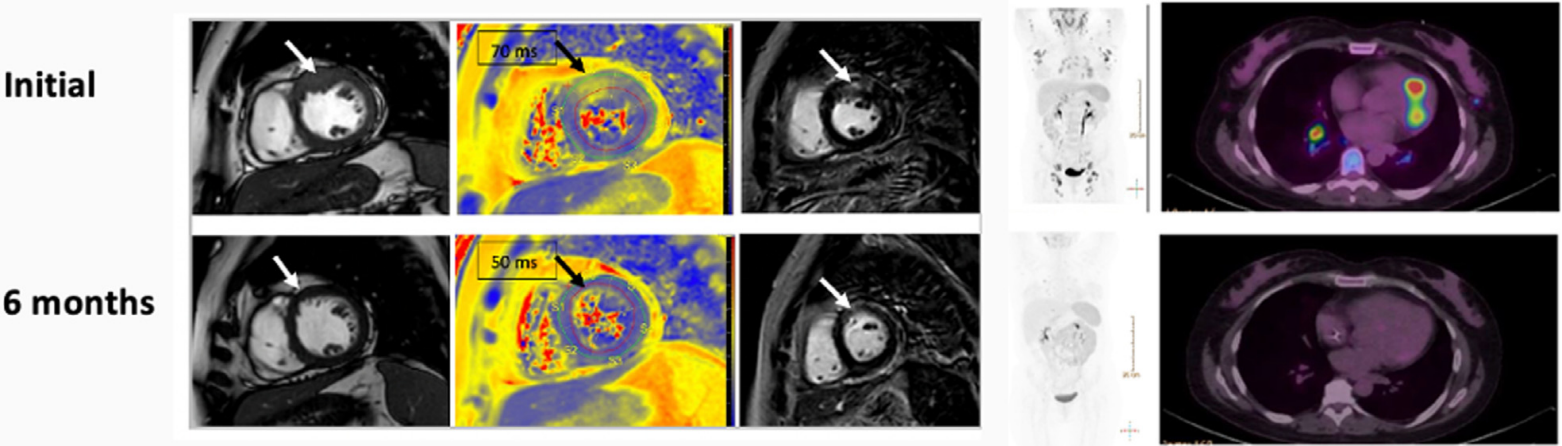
Comparison of Initial and Follow-Up Imaging (Top left) Initial cardiac magnetic resonance (from left to right: cine, T2 mapping, and late gadolinium enhancement sequences) and (top right) initial fluorine-18 fluorodeoxyglucose positron emission tomography/computed tomography scans. (Bottom left) Follow-up cardiac magnetic resonance and (bottom right) follow-up fluorine-18 fluorodeoxyglucose positron emission tomography/computed tomography scans. The arrows point out the inflamed segment that heals with chemotherapy.
